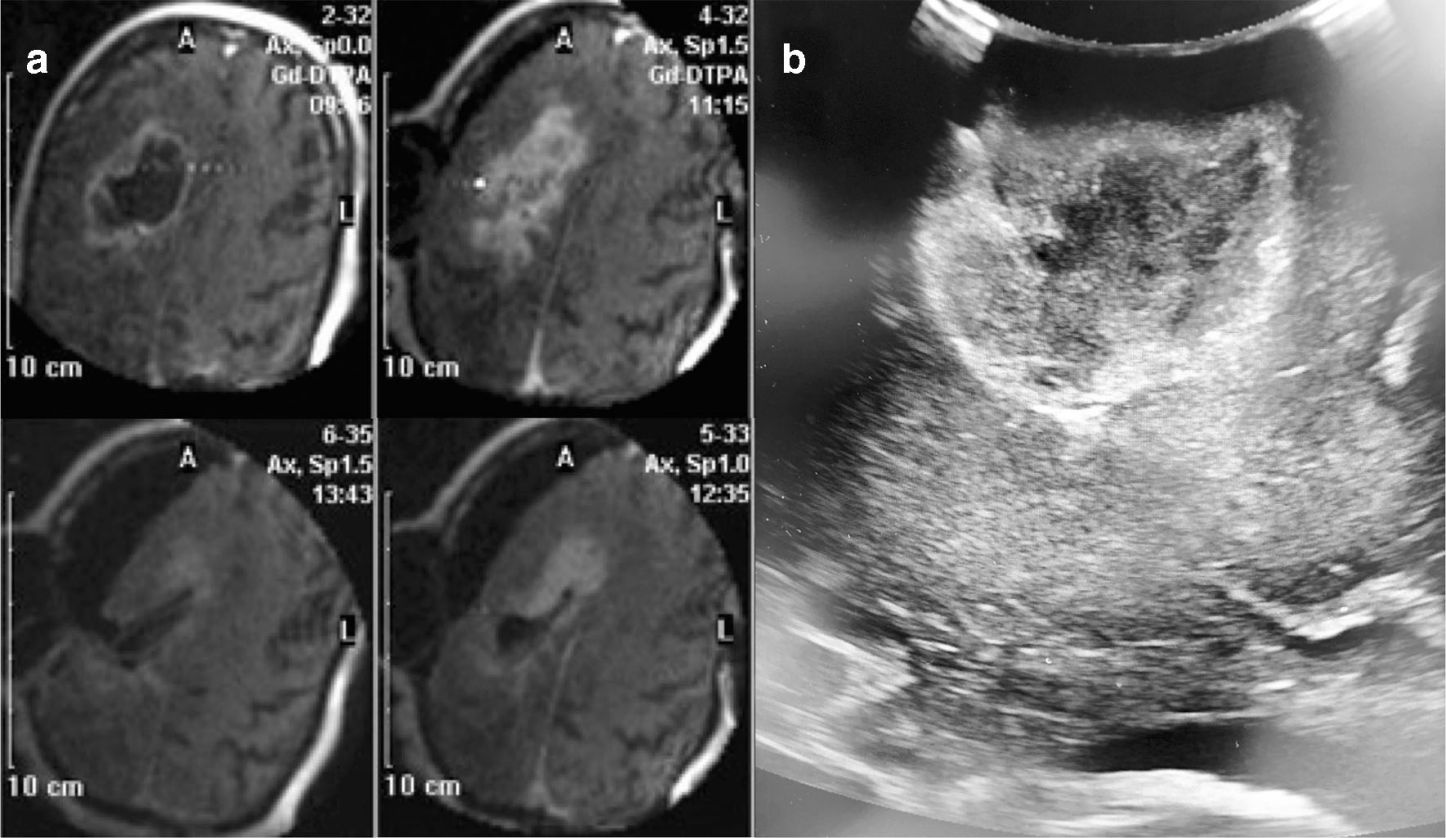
Examples of intraoperative imaging. A Intraoperative MRI of an enhancing right frontal glioblastoma with clockwise images of the progression of the resection with clear brain shift. Image courtesy of Dr. P Kubben [46]. B Intraoperative ultrasound of a left parietal glioblastoma
